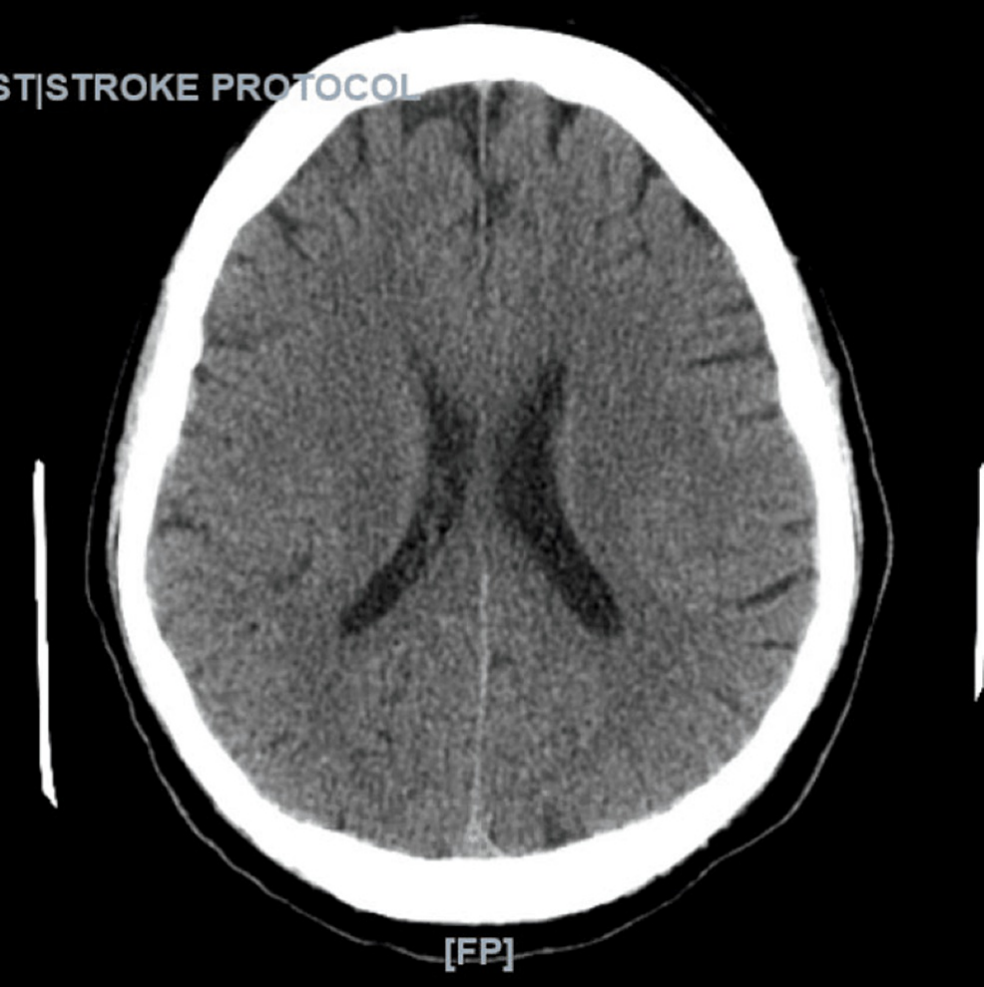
CT of the head without contrast (axial view) was negative for intracranial hemorrhage. There was no evidence of an acute cortical infarct, mass effect, midline shift or, hydrocephalus.
